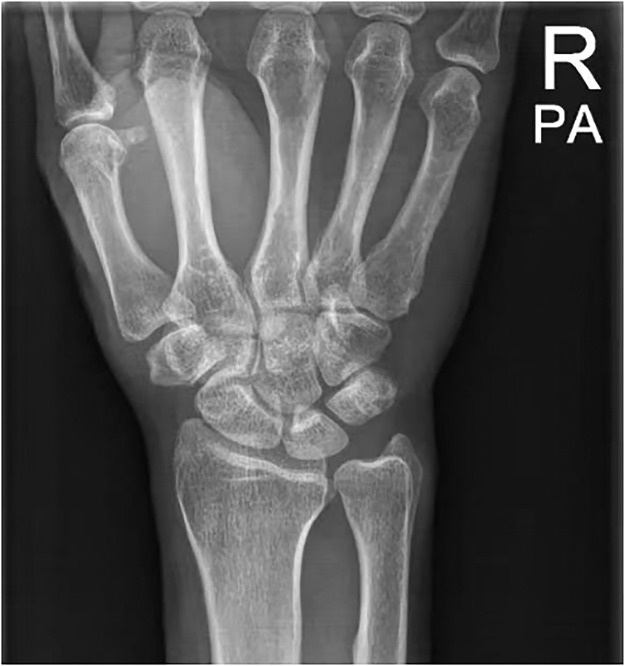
After treatment radiographic findings. X-ray image demonstrating complete debridement of calcific deposits.
